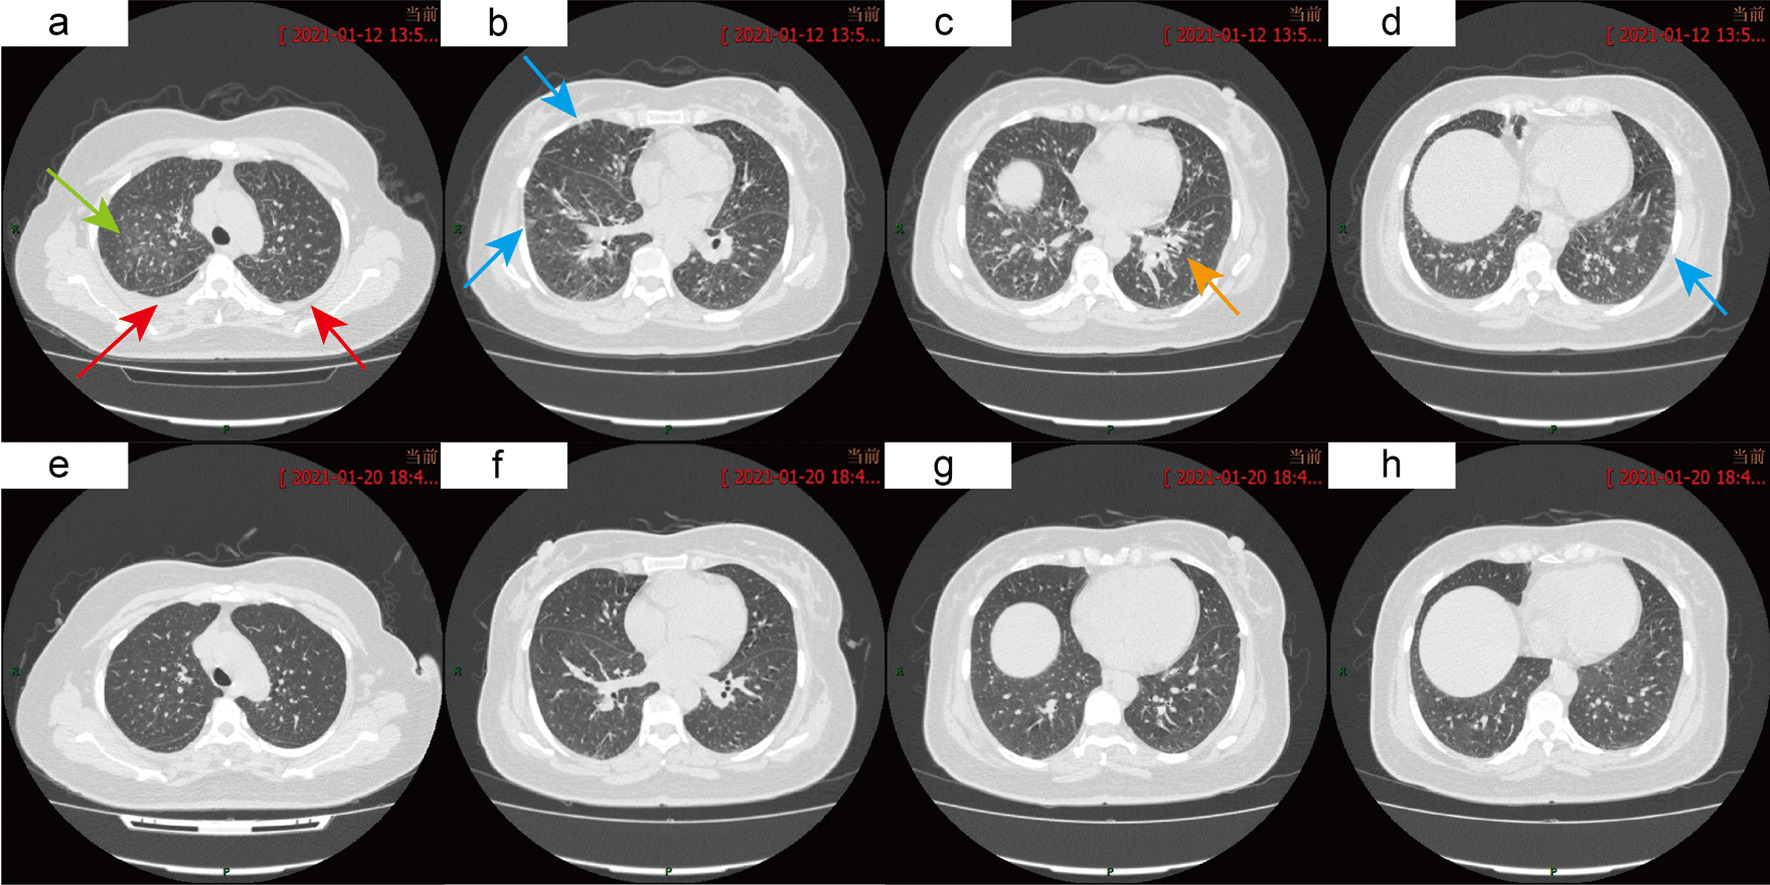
Changes in the chest CT (a–d are on D13: random pulmonary nodules (green arrow), a small amount of bilateral pleural effusion (red arrows), multiple areas of ground glass opacity (blue arrows), thickening of bronchovascular bundles (orange arrow); e–h are on D21: radiological improvement after withdrawal of the drug.)
